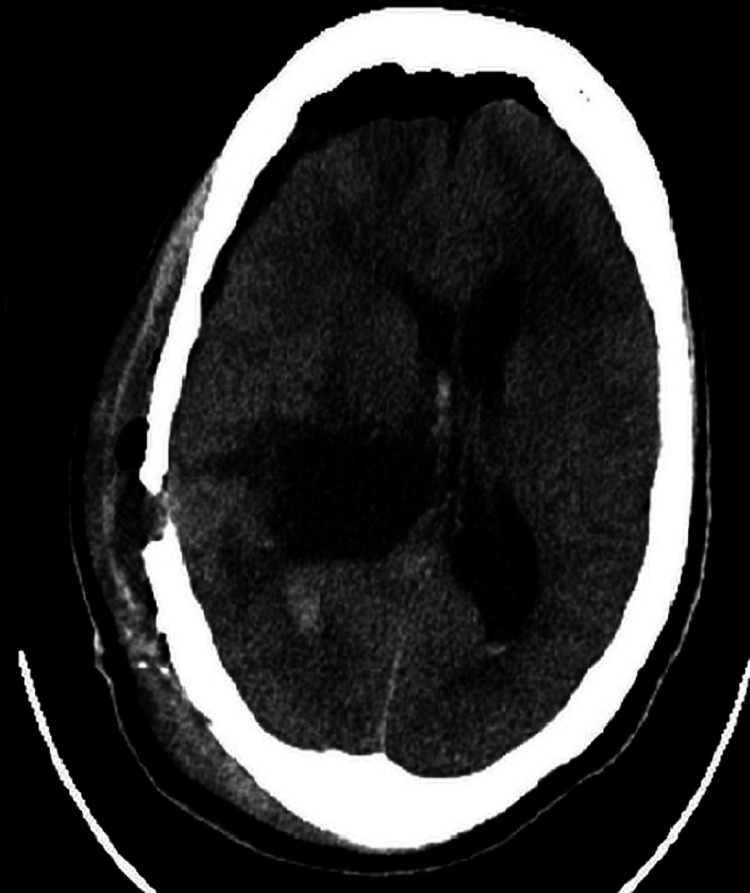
CT brain non-contrast demonstrating resection of tumour within the right lateral ventricle, with post-surgical changes.
